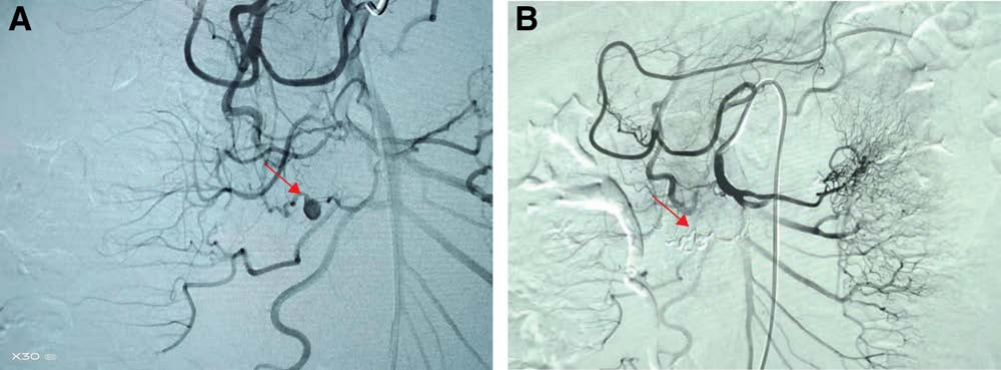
(A) Superior mesenteric artery for angiography showed a pseudoaneurysm at the pancreaticoduodenal arch. (B) The angiography showed that the pseudoaneurysm was occluded, and the distal cavity was not redeveloped. (C) CT of the upper abdomen showed what was seen after celiac artery embolization.
